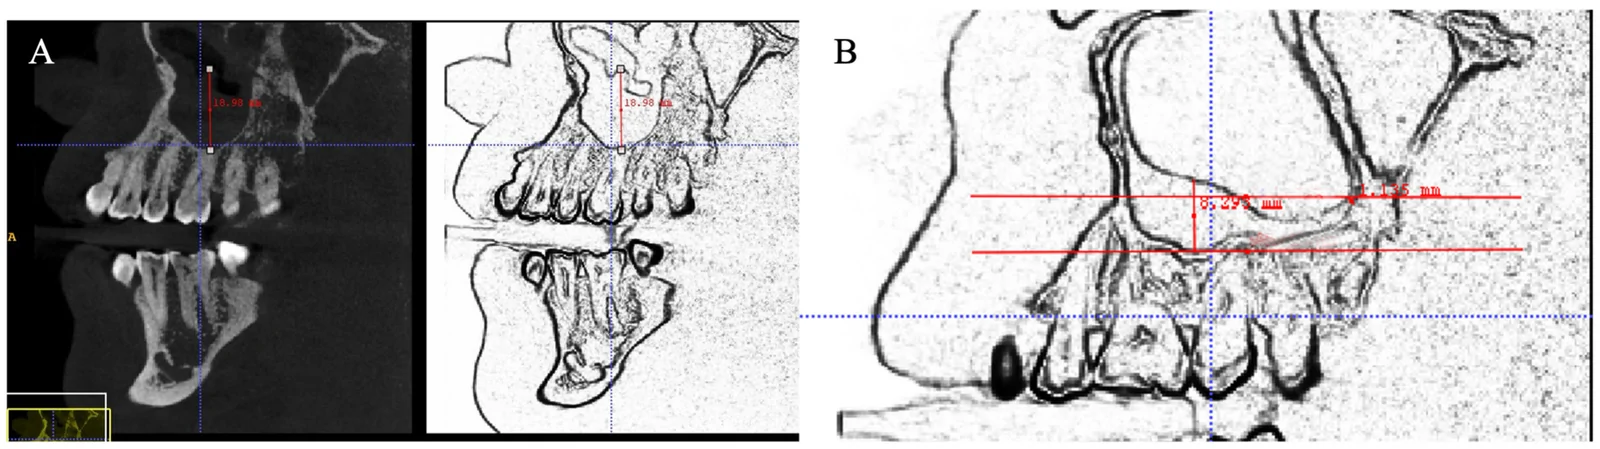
Coronal CBCT views of maxillary sinus demonstrating Schneiderian membrane thickening. (A) Extensive Schneiderian membrane thickening occupying nearly the entire maxillary sinus cavity. (B) Localized Schneiderian membrane thickening.
